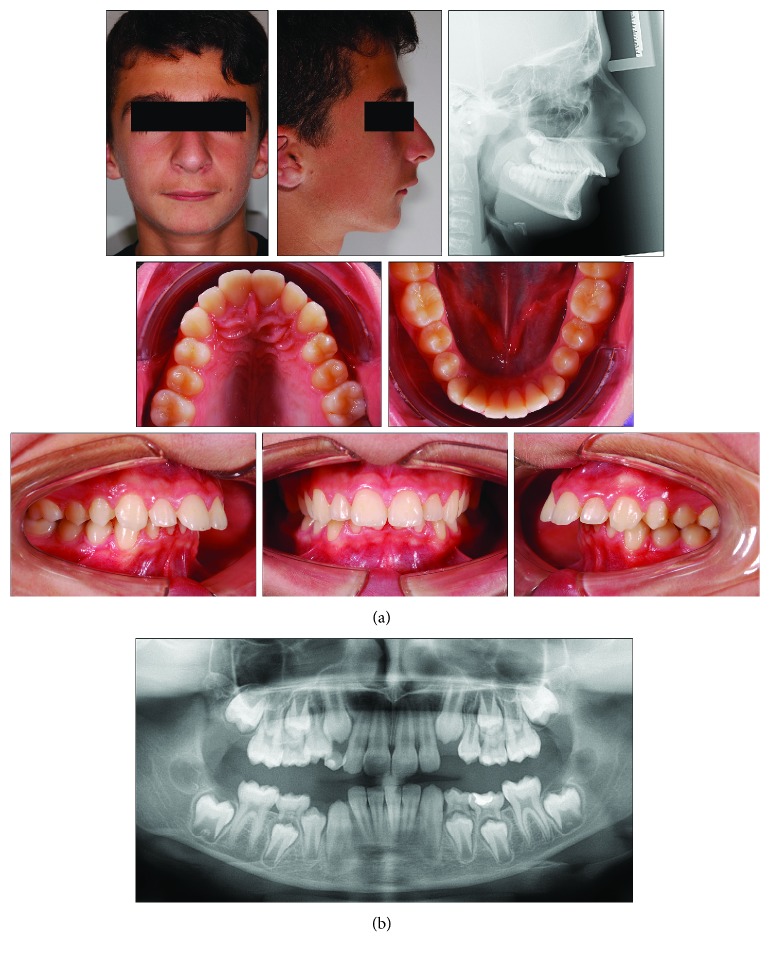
Case 1. (a) 9-year and 10-month-old male patient with skeletal Class II malocclusion, increased overjet, and overbite before treatment. (b) Panoramic radiograph taken at first visit when the patients was 9 years old.
